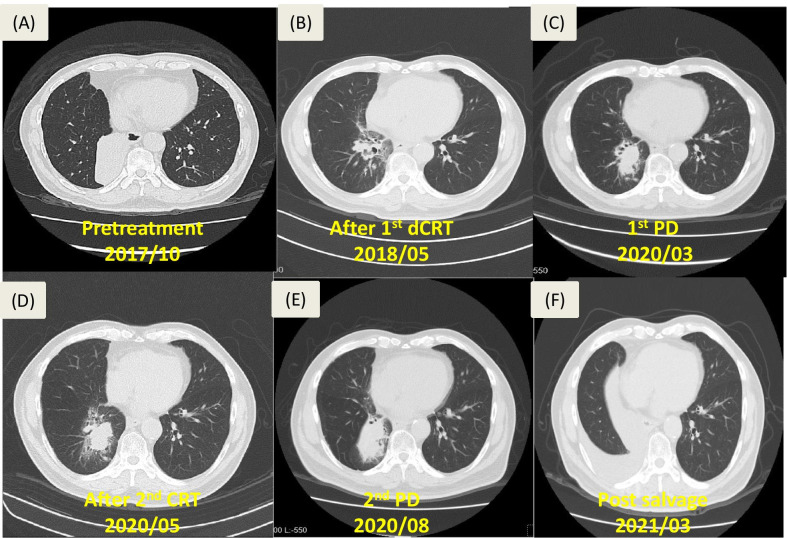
Series of chest CT scan images showing the tumor morphology from pre-treatment to pre-operative state. a Before 1st dCRT on 2017/10/16; b after the 1st dCRT on 2018/05/15; c post-dCRT surveillance showing tumor recurrence on 2020/03/04; d after the 2nd dCRT on 2020/05/18; ebefore salvage resection on 2020/08/18; f surveillance on 2021/03/22, 6 months after the surgery
